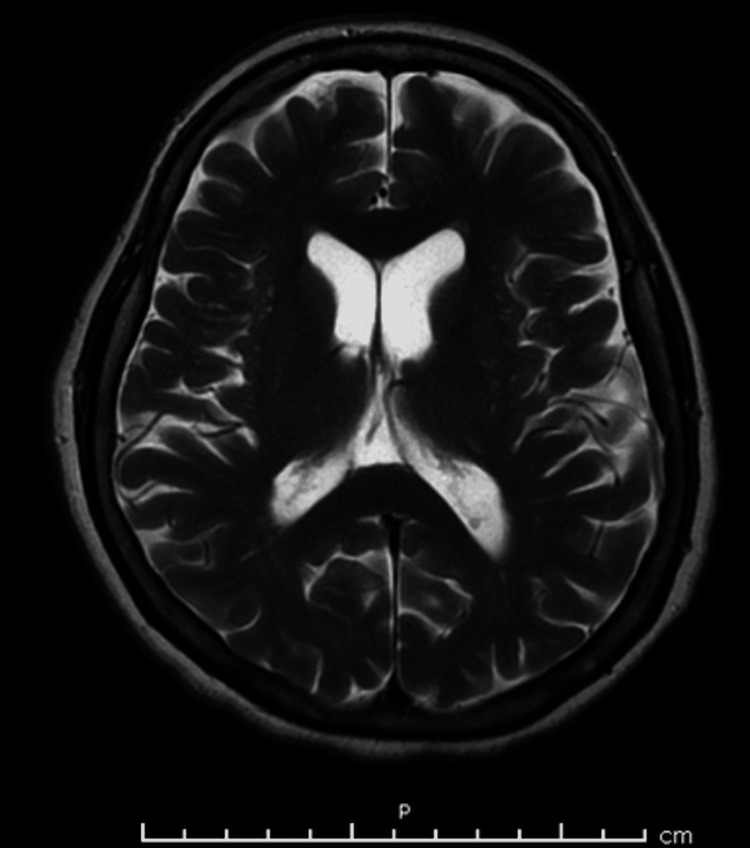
MRI of the brain showing no signs of acute bleeding and/or ischemia (plane B).
